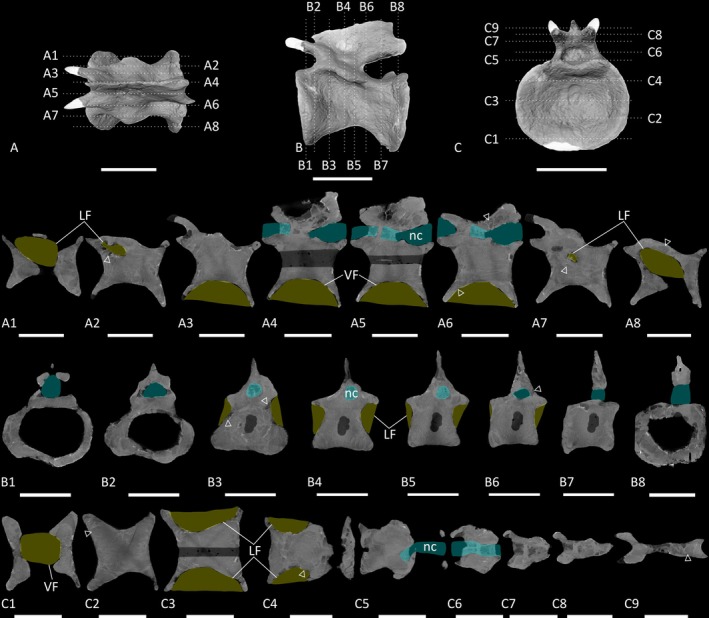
CT scan of *Giraffatitan brancai* caudal vertebra 19 MB.R.5000.18. (A–C) 3D rendering (A) dorsal view, (B) left lateral view, (C) anterior view; (A1–A8) CT scan slices in parasagittal cross‐section, (B1–B8) CT scan slices in transverse cross‐section, (C1–C9) CT scan slices in horizontal cross‐section. LF, lateral fossa; nc, neural canal; VF, ventral fossa. Key: Blue highlight, neural canal; yellow highlight, centrum fossa; white arrow, pneumatic bone texture. Scale bars = 100 mm.
